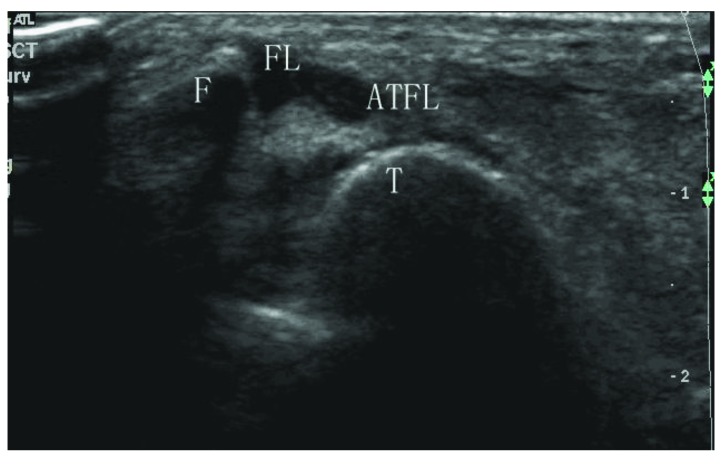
Sonogram shows a total tear at the fibular side (type IIB). ATFL: anterior talofibular ligament; F: fibula; T: talus; FL: fluid.
